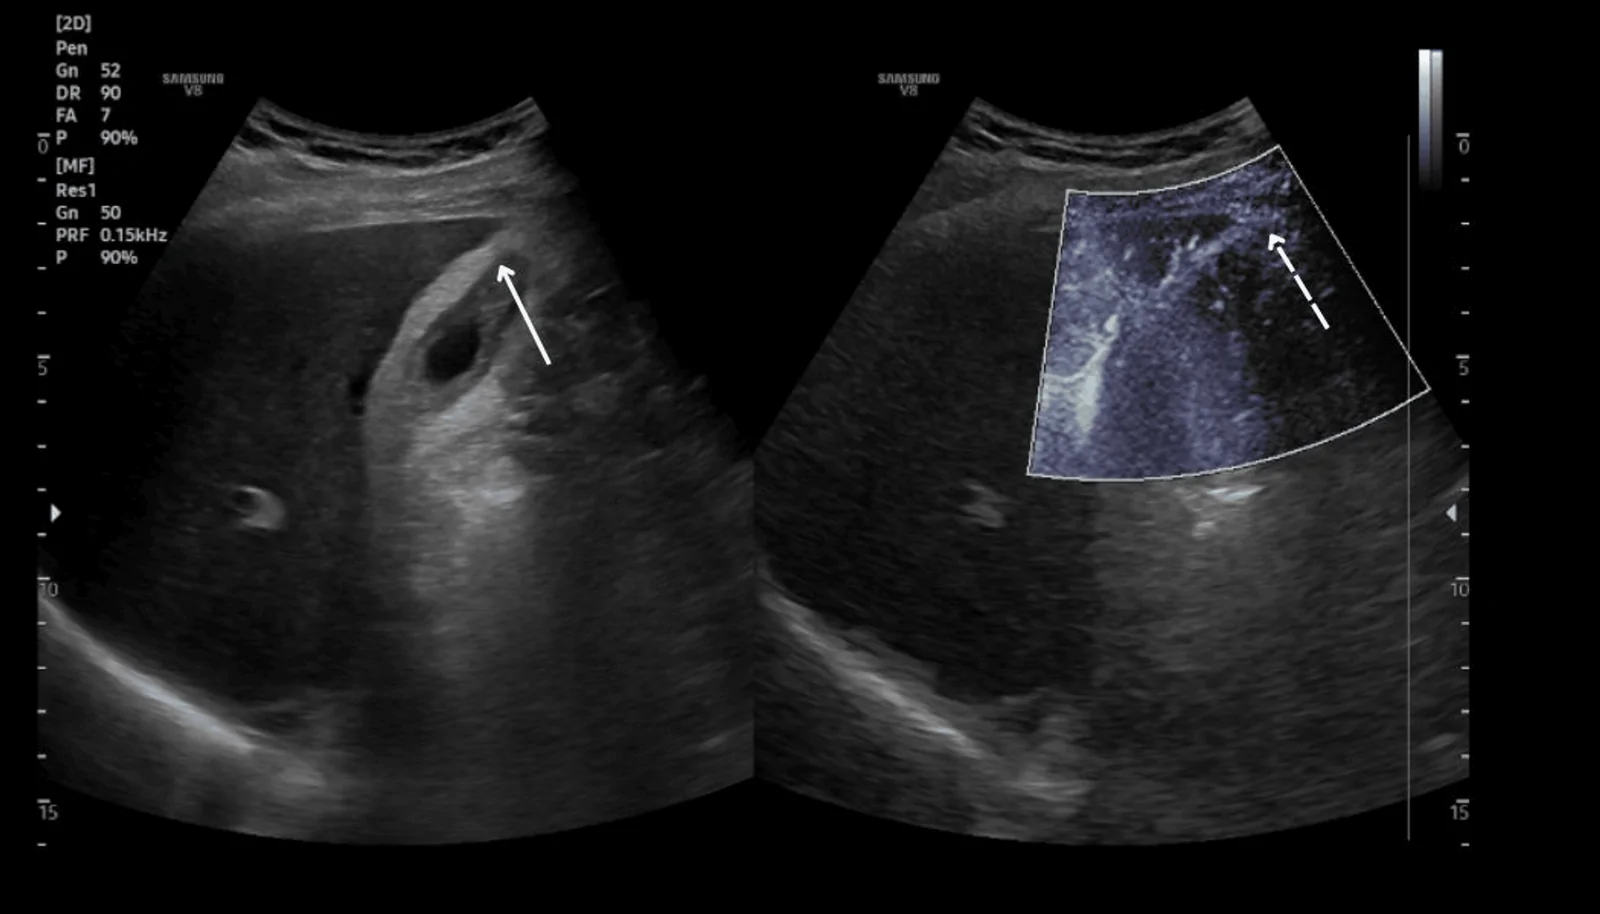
B-mode ultrasound image (curvilinear probe) showing focal wall thickening (left) and microvascular flow showing no increase in vascularity (right) Left: arrowhead pointing toward focal wall thickening at the fundus of the gallbladder. Right: dotted arrowhead pointing toward the fundus of the gallbladder, showing no increase in vascularity.
